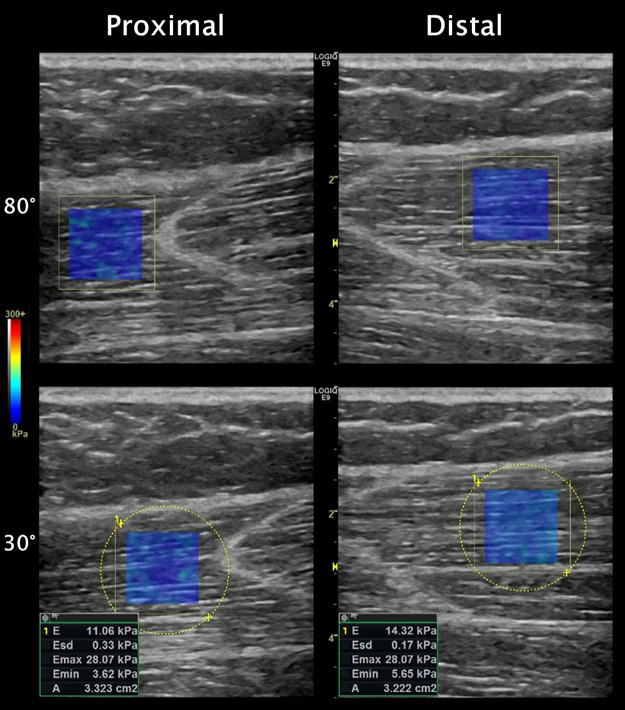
Exemplary shear-wave elastography images of the proximal (*left*) and distal (*right*) compartments of semitendinosus at 80° (*upper*) and 30° (*lower*) of knee joint flexion. The colored rectangle within each ultrasound image is the elastography map, with the scale (Young’s modulus) on the *outer left* of the figure. Note the similarities between ultrasound image features despite repositioning the transducer over the skin for every measurement (without the aid of indelible ink markings). The tendinous inscription is visible as the hyperechoic V-shaped structure adjacent to the elastography color maps. Examples of the measurement method using the internal software of the device are shown on the lower images.
